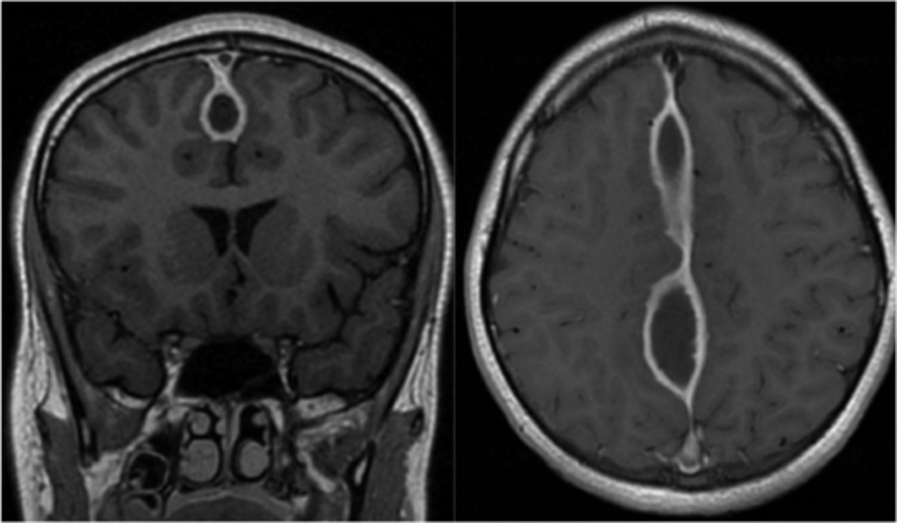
Interval MRI (one month later) showing enlargement and dural thickening of prior empyema
